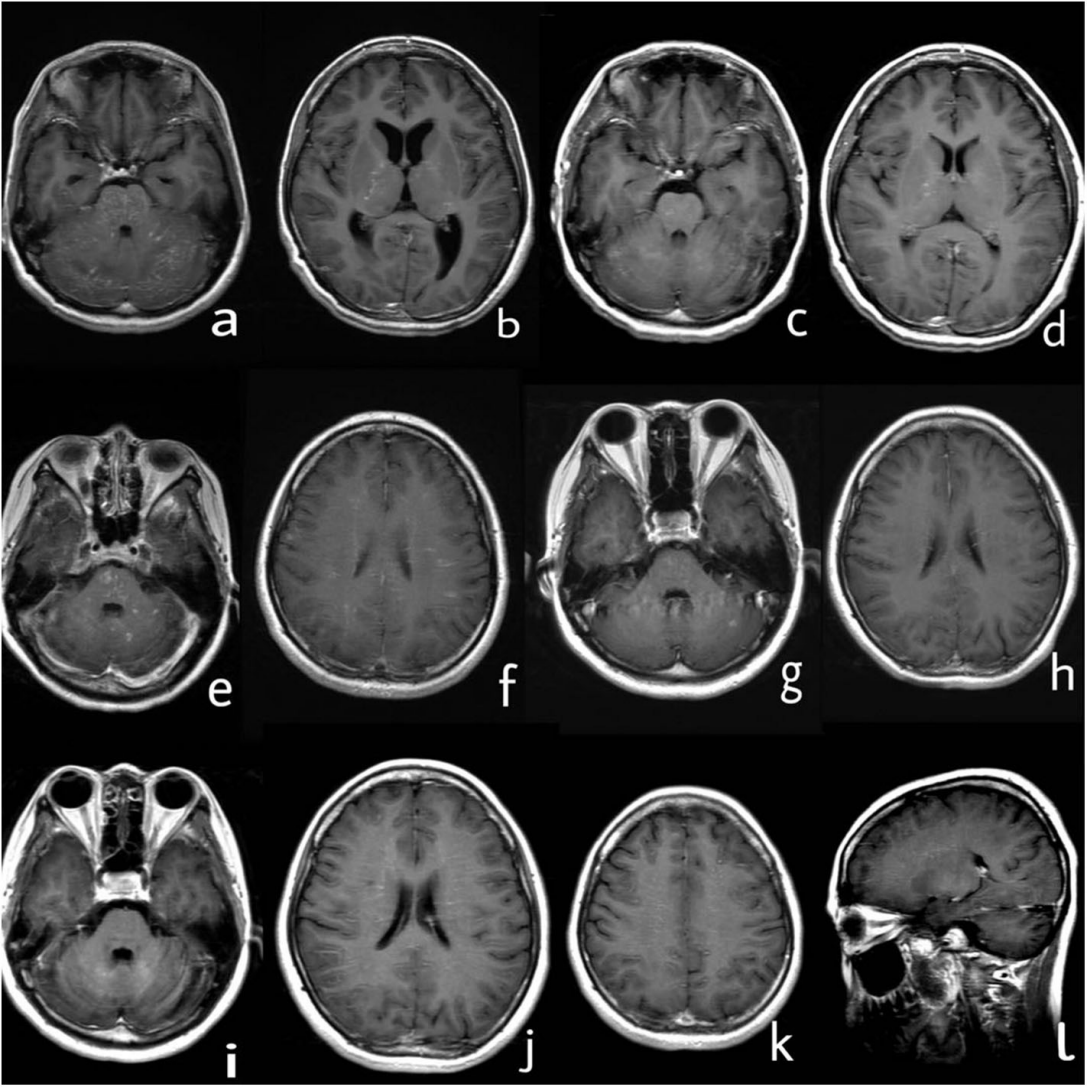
Series of brain MRI with contrast. (a,b) In March 2012, curvilinear and punctate enhancements of pontine, cerebellum, and basal ganglion were demonstrated. (c,d) In June 2012, enhancement of pontine, cerebellum, and basal ganglion improved after treatment with steroids. (e,f) In June 2015, novel dot-like and liner enhancement in pontine, semiovale center, and juxtacortical area were shown. (g,h) In September 2015, enhancement in pontine, semiovale center, and juxtacortical area improved again after treatment with steroids. (i–l) In September 2019, novel dot-like and liner enhancement in both hemispheres of the cerebellum, semiovale center, basal ganglion, and thalamus were demonstrated.
